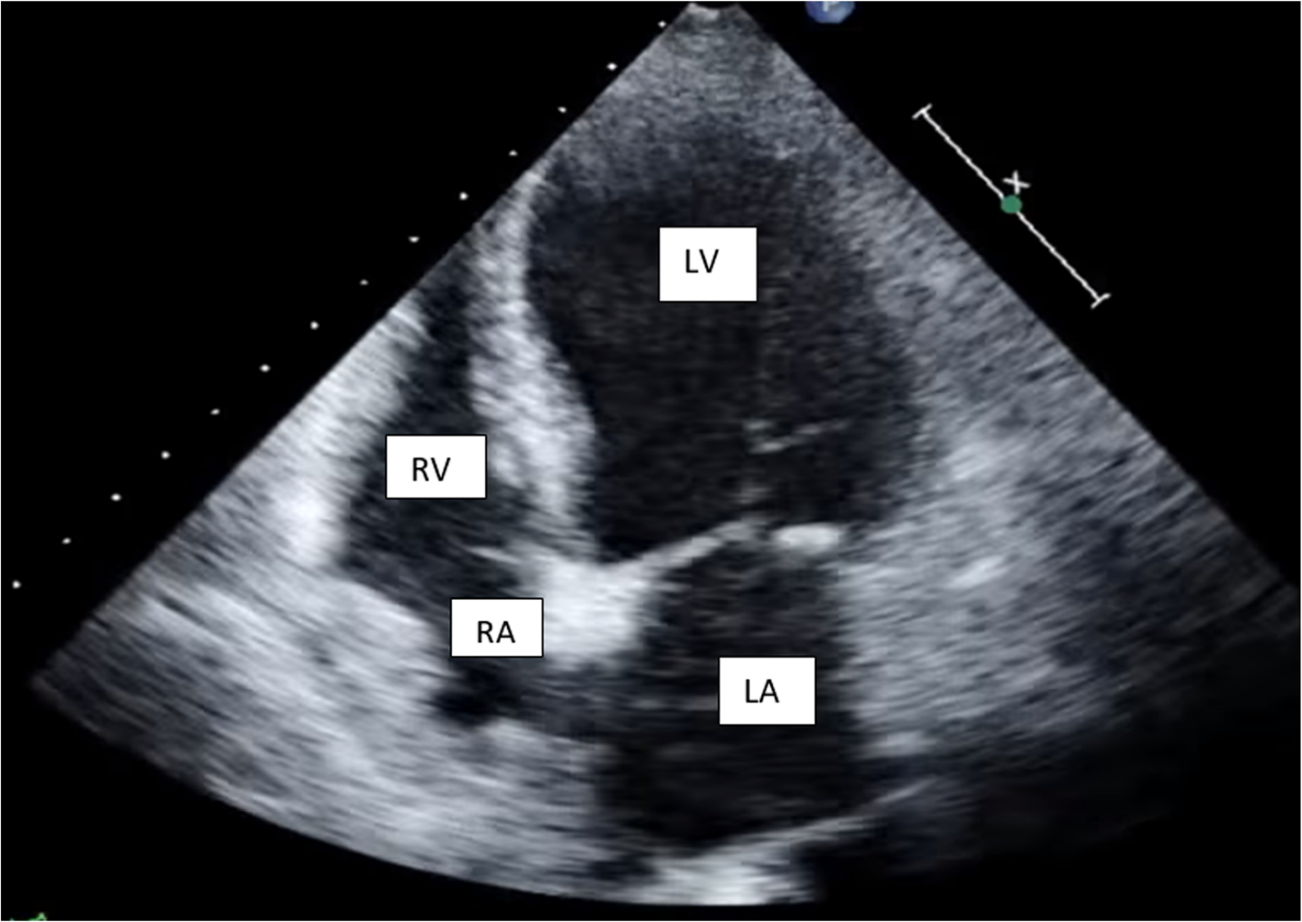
Transthoracic echocardiography showing apical ballooning of the left ventricle (LV: Left Ventricle; RV: Right Ventricle; LA: Left Atrium; RA: Right Atrium)
